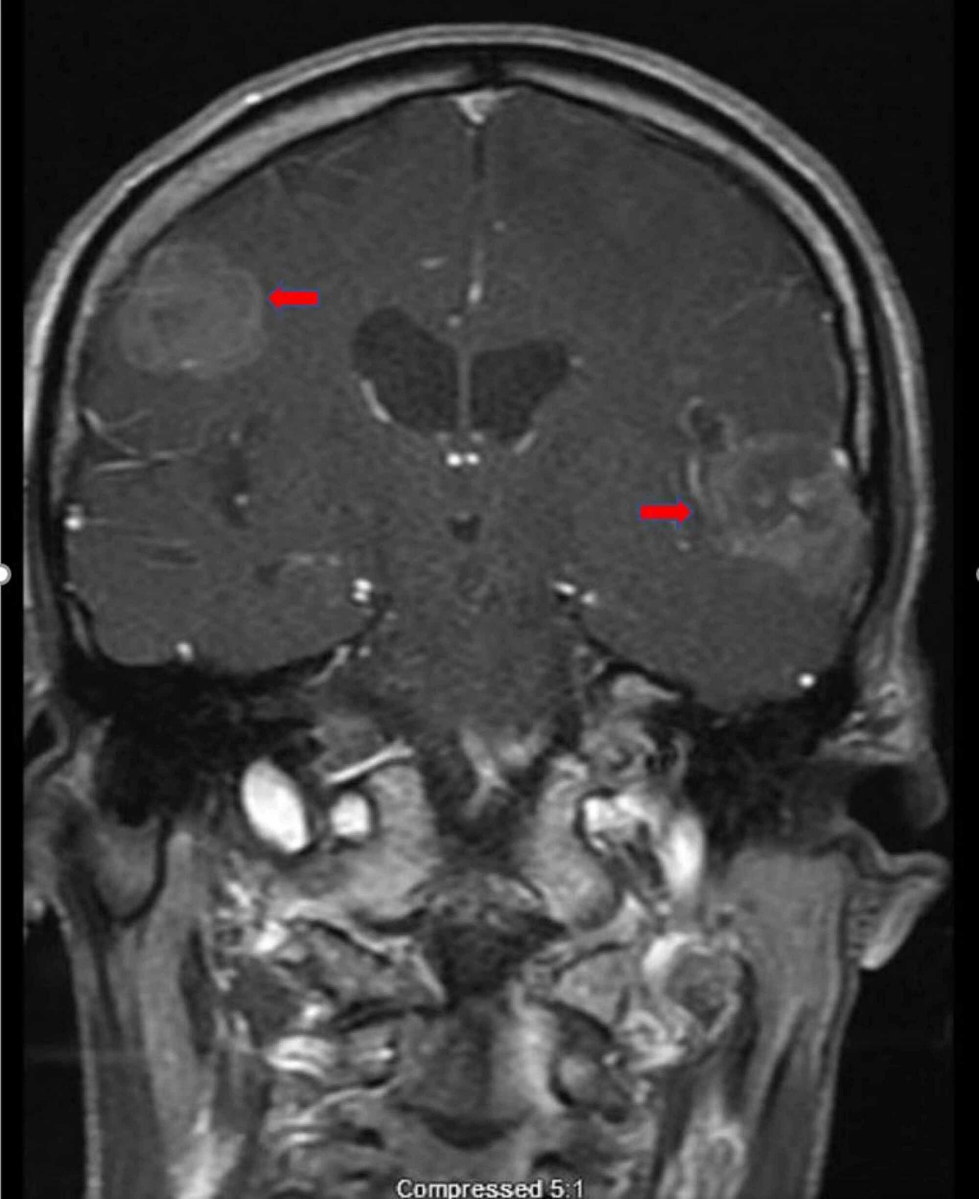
MRI brain with contrast, coronal section. Red arrows showing metastatic lesions.
